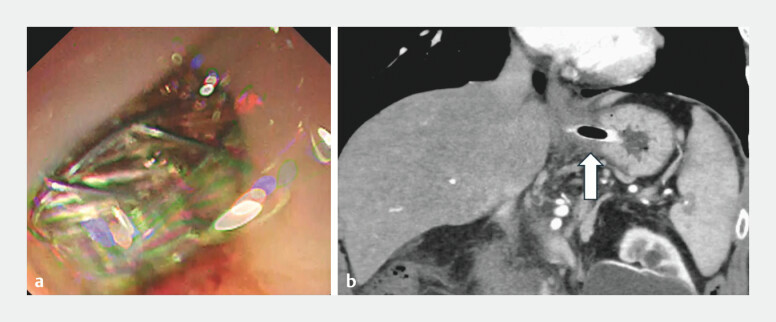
The position of the self-expanding metal stent (SEMS) after endoscopic ultrasound-guided hepaticogastrostomy.
